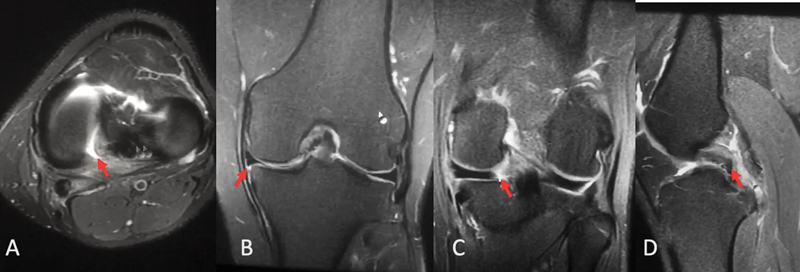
Magnetic resonance imaging scan of a patient with posterior meniscal root injury. (
